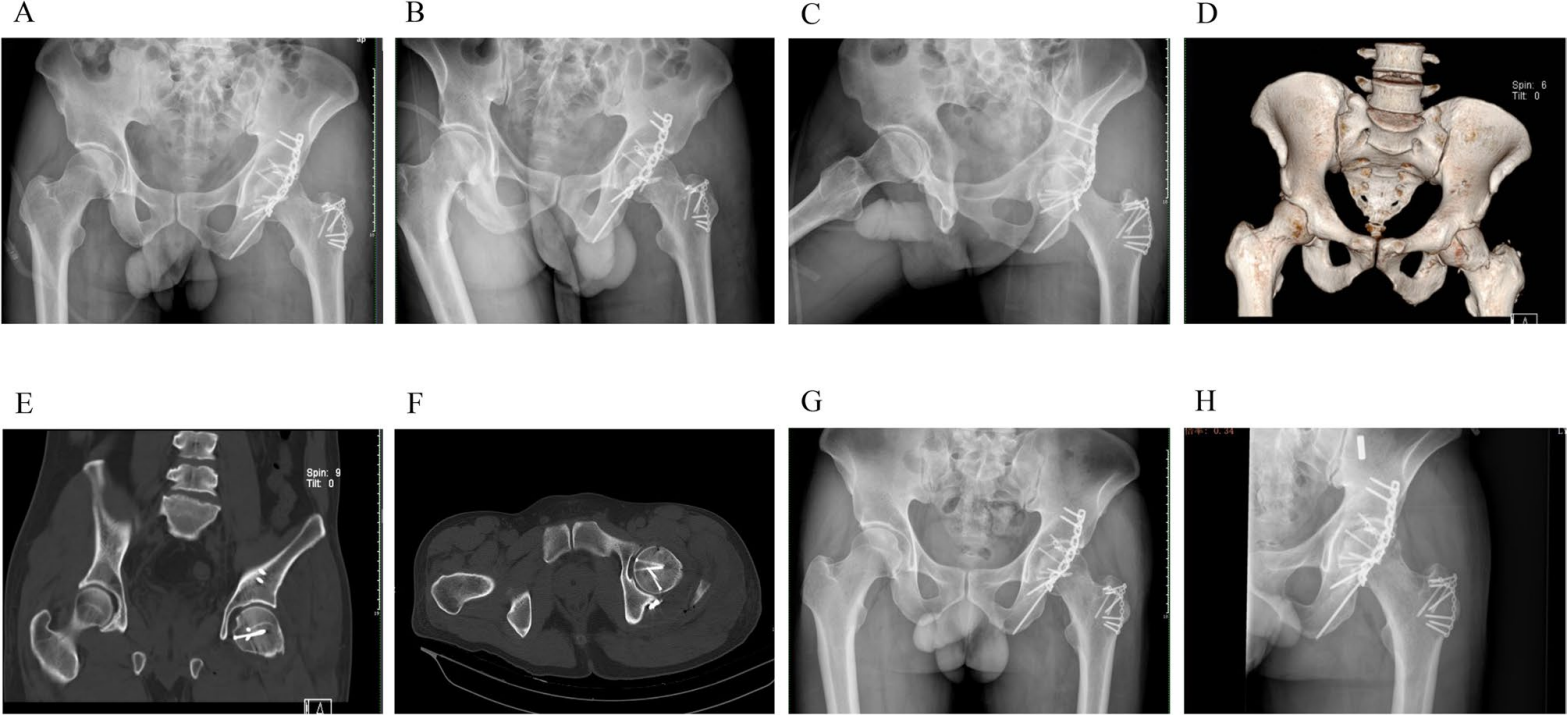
The X-ray and CT images of this male patients A-C: The X-ray film on the second day after surgery showed that the acetabular fracture was anatomic reduction, and the reduction quality of the femoral head fracture was excellent D-F: CT scan on the second day after surgery indicated that the acetabular fracture was anatomic reduction, and the reduction quality of femoral head fracture was excellent G: Radiographs 1 month after surgery showed that femoral head fractures and acetabular fractures were in good position and internal fixation was firm H: Radiographs 3 months after surgery showed that fractures of the femoral head and acetabulum were healed and internal fixation was firm
